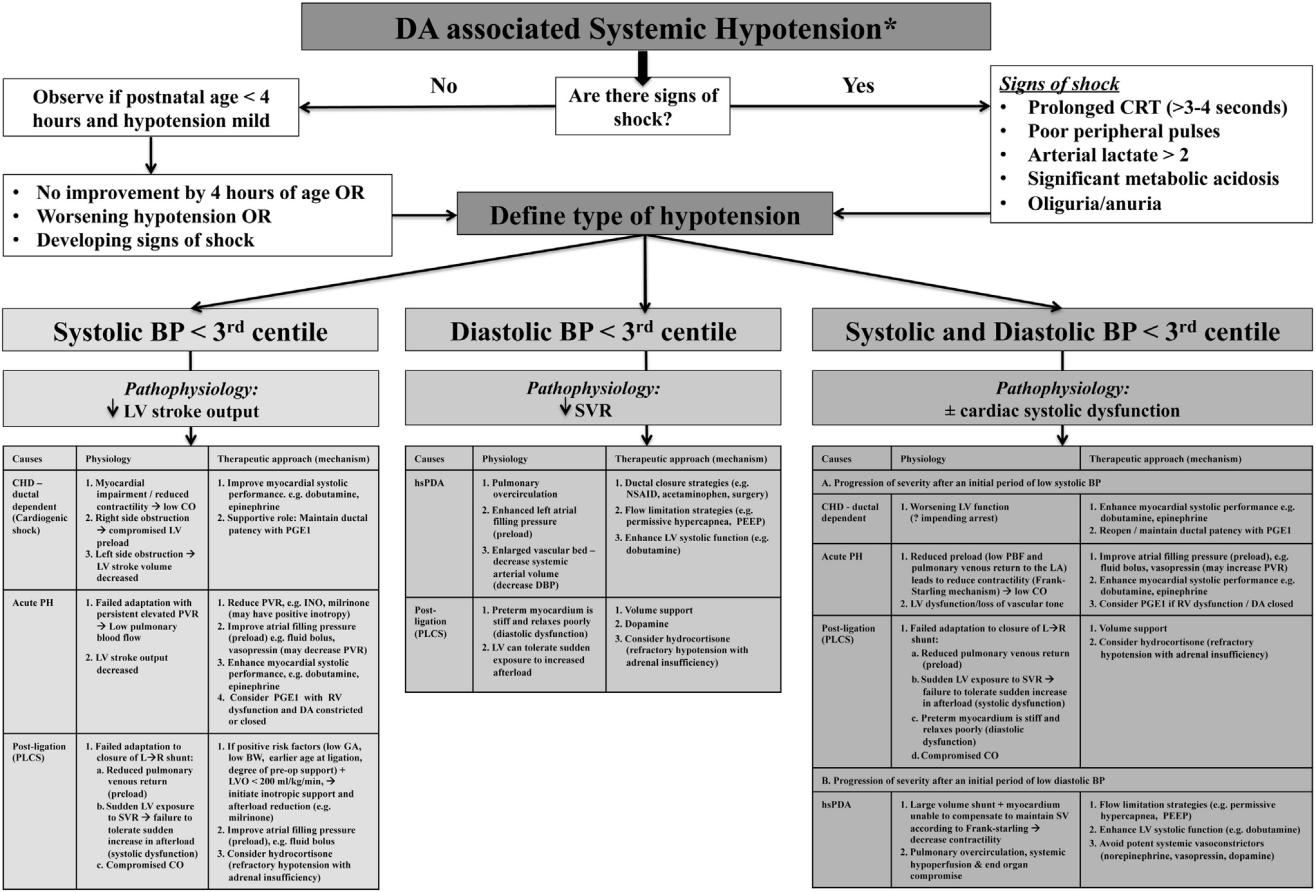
Algorithm for the assessment and treatment of ductus arteriosus (DA)-associated hypotension according to systolic, diastolic, and combined systolic and diastolic categories. *Global assessment of systemic perfusion from integration with arterial pressure thresholds may provide early clinical insights regarding pathophysiologic determinants of hemodynamic instability, but ascertainment and confirmation of the presence of hemodynamics significance must be obtained using comprehensive echocardiography.
